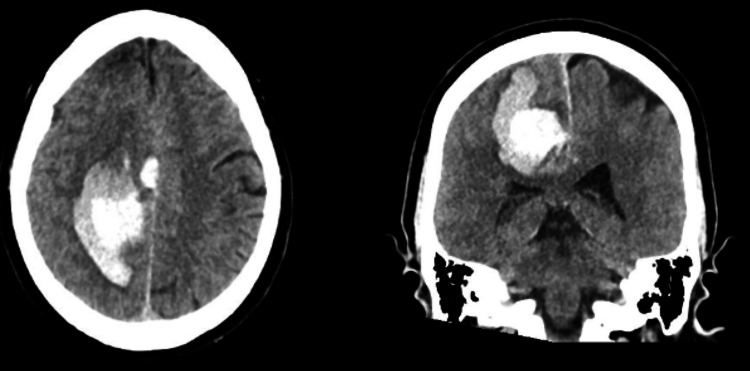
Preoperative CT scan for patient 2 Preoperative CT scan with a significant right frontal sICH sICH: Spontaneous intracerebral hemorrhage
